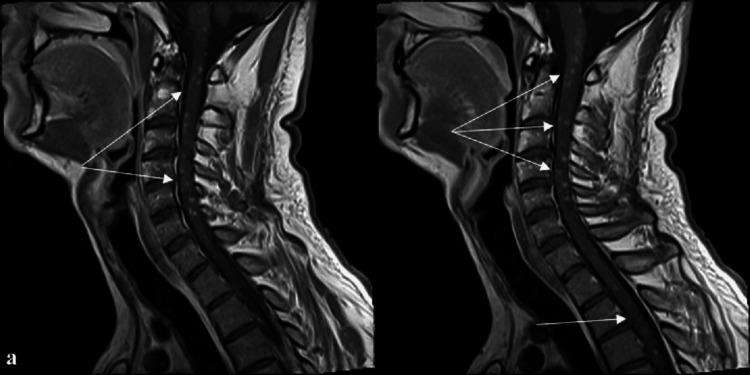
Sagittal T1 MRI of the spine showing multiple round hyperintensities in the cervical and upper thoracic spinal cord MRI: magnetic resonance imaging
